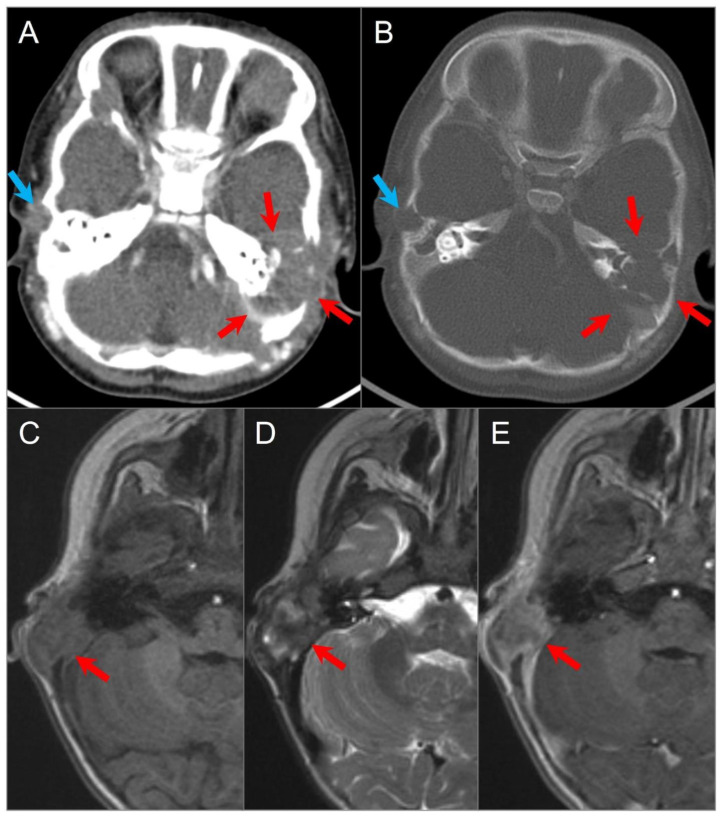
Langerhans cell histiocytosis in a 9-month-old boy. Axial postcontrast CT in soft tissue (A) and bone window (B) settings shows a lytic ‘punched out’ bone lesion involving the left petromastoid temporal bone with middle and posterior cranial fossa extensions (red arrows). The lesion also erodes the left semicircular canals and involves the left middle ear cavity and facial nerve. Another small destructive lesion with similar CT characteristics is seen in the right temporal bone (blue arrows). In a different 3-year-old boy, (C) noncontrast T1W, (D) T2W, and (E) postcontrast T1W axial images demonstrate a relatively well-delineated lesion in the right temporal bone (red arrows). The lesion demonstrates a slightly heterogeneous isointense signal on T1 and mixed hypointense and hyperintense signals on T2WI with heterogeneous enhancement after gadolinium administration.
